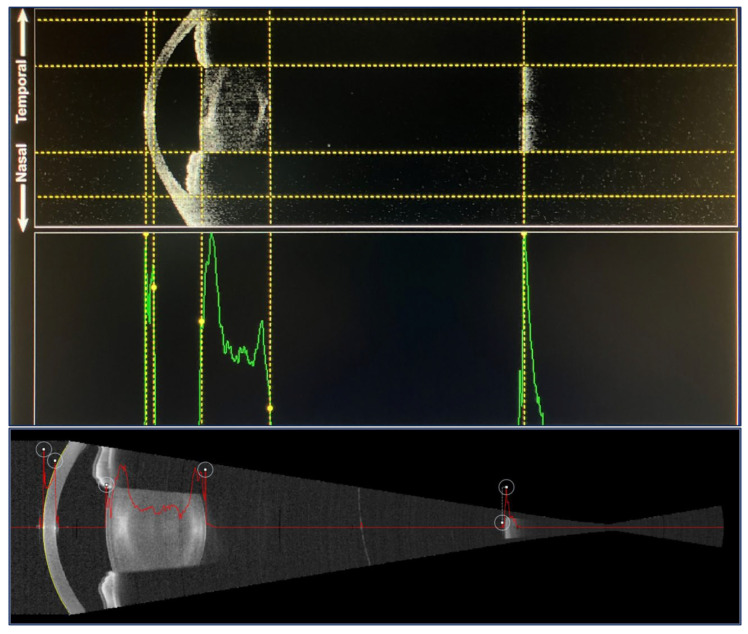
Example of swept-source optical coherence tomography B-scan biometry image of the anterior segment up to the posterior pole with each device. Top, Argos^®^ biometer. Yellow vertical lines represent the boundaries of each intraocular structure, while green lines are the intensity of the ultrasound signal in the A-scan. Bottom, Eyestar 900^®^ biometer. The red lines represent, again, the intensity of the ultrasound echo in the A-scan. In each image and from left to right, the different hyperreflective structures represent the anterior and posterior surfaces of the cornea, the anterior and posterior surfaces of the lens, and finally the retina. The hyporeflective space between the cornea and the lens represents the anterior chamber and the corresponding structure between the lens and the retina is the vitreous cavity. The group refractive index assigns a single mean n to the whole eye to derive the axial length; the sum of segments approach assigns *n* = 1.376 to the cornea, *n* = 1.336 to the aqueous and the vitreous humor, and *n* = 1.410 to the lens.
